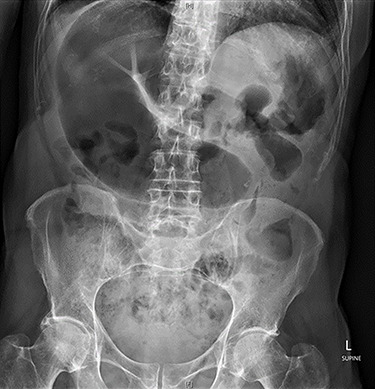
Abdominal X-ray on presentation showing a distended loop of colon with air-fluid levels.
